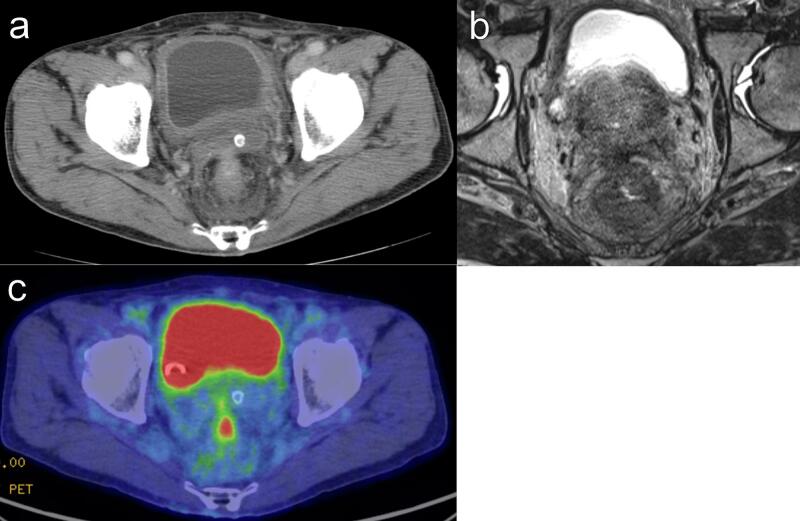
Radiological and colonoscopy findings before total pelvic exenteration. A) Abdominal contrast-enhanced computed tomography showing significant reduction in rectal wall thickness. B) Pelvic magnetic resonance imaging showing no perirectal abscess and an indistinct border between the tumor and the prostate. C) ^18^F-fluorodeoxyglucose (FDG)-positron emission tomography/computed tomography showing a reduction in FDG uptake and absence of distant metastasis.
